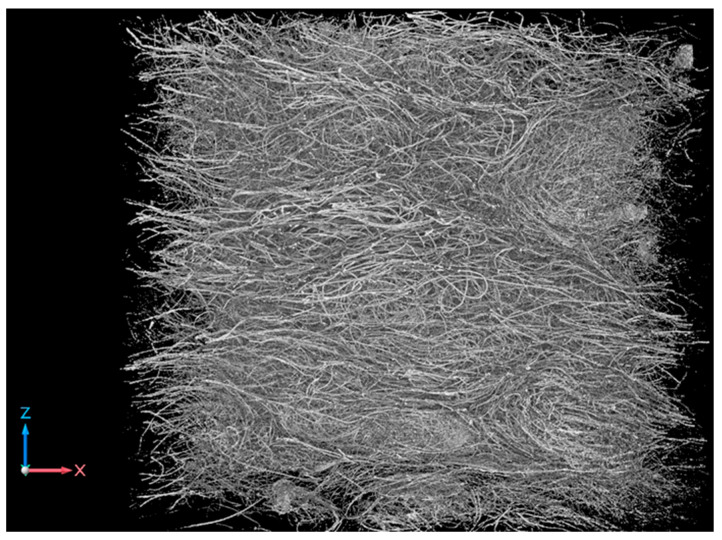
3D image from X-ray computed tomography (CT) of insulator No. 4 with irregular structure and low fibre orientation.
